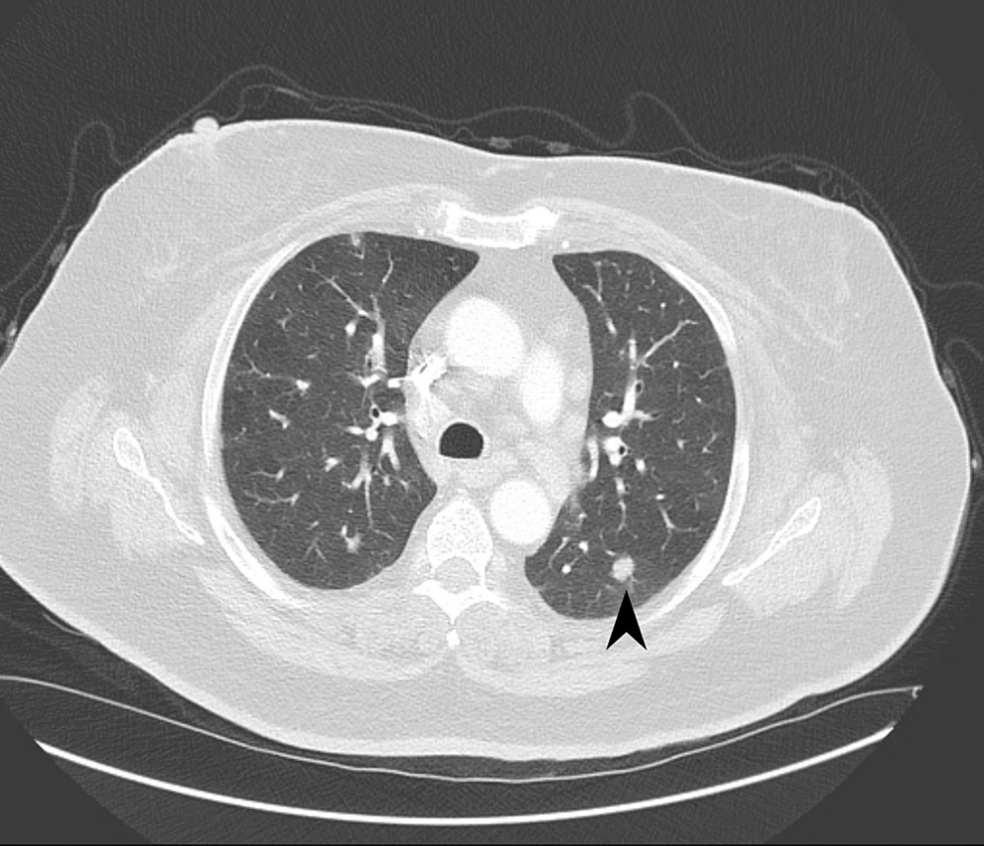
Transverse section of CT scan of the chest demonstrating large pulmonary nodule in the left posterior, upper lung field that was targeted for biopsy (black arrowhead).
